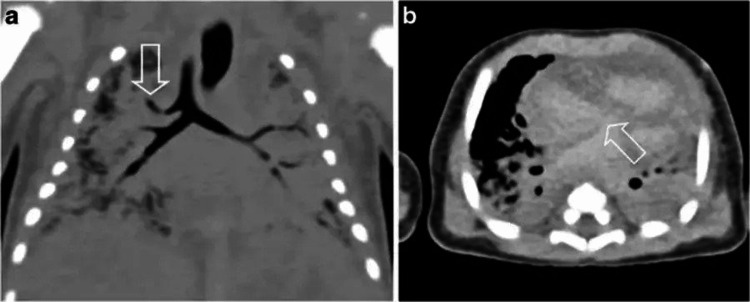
Example of postmortem CT scan in a five-day-old boy: (a) tracheal right upper lobe bronchus is seen via arrow on coronal reconstruction. (b) Atrial septal defect is seen via arrow on axial image.
